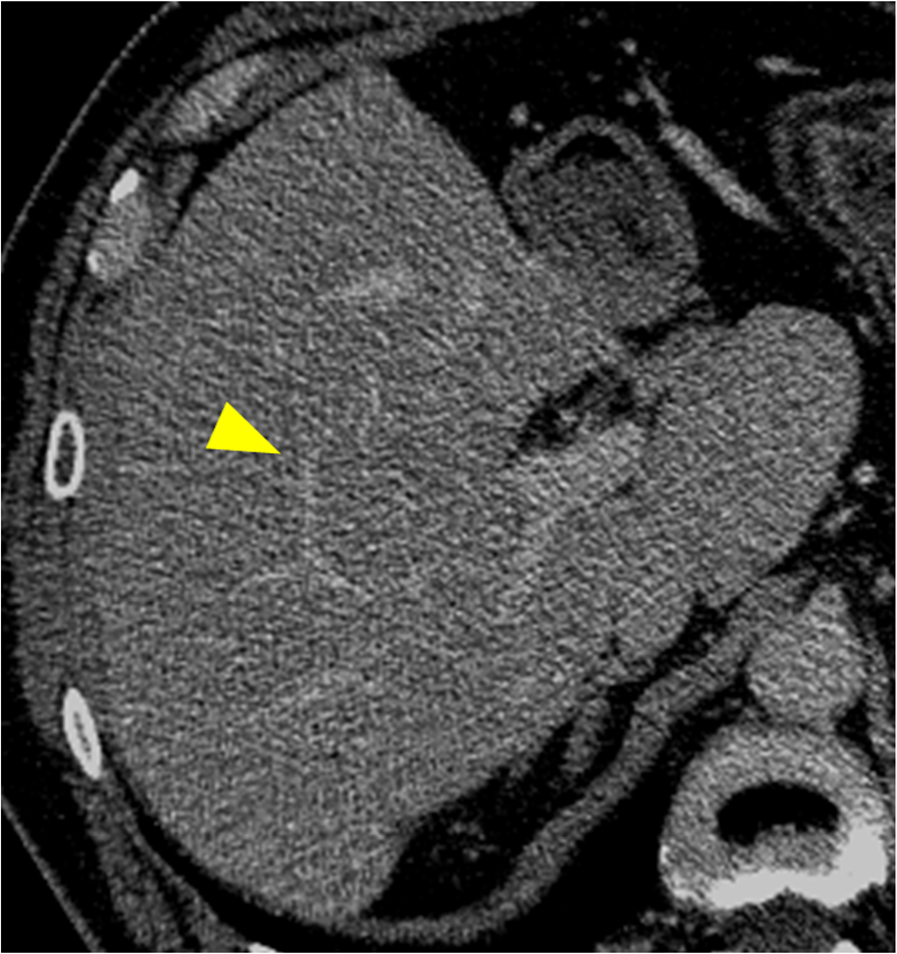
Contrast-enhanced CT performed 10 months after the second hepatectomy. Intrahepatic venous collaterals (*yellow arrowhead*) between the RHV and MHV remained patent
